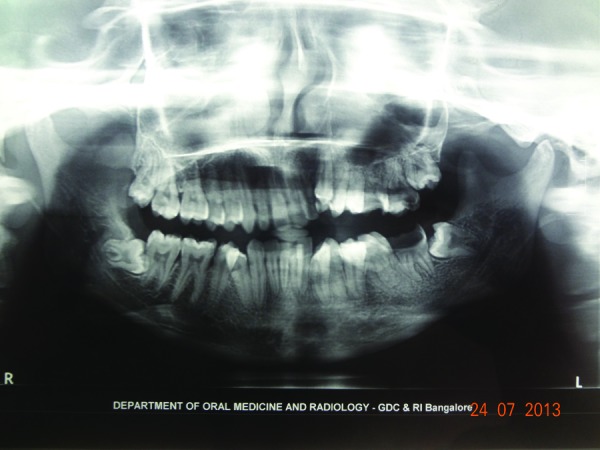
Panoramic Radiograph showing asymmetry with smaller condyle and coronoid process, short roots on affected side when compared to right side.
